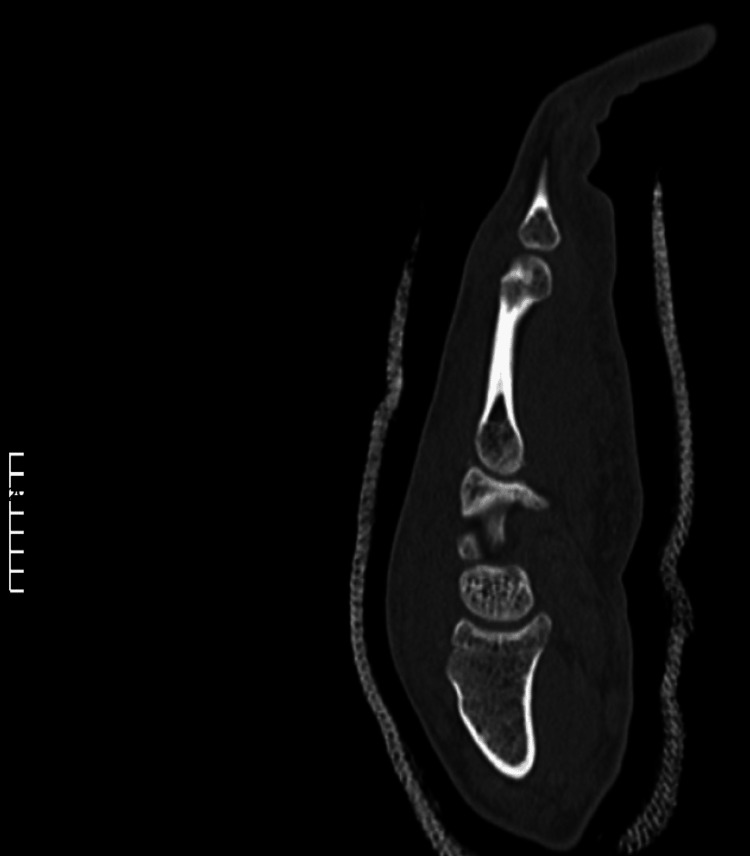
Post-reduction lateral CT view of the carpometacarpal joint
